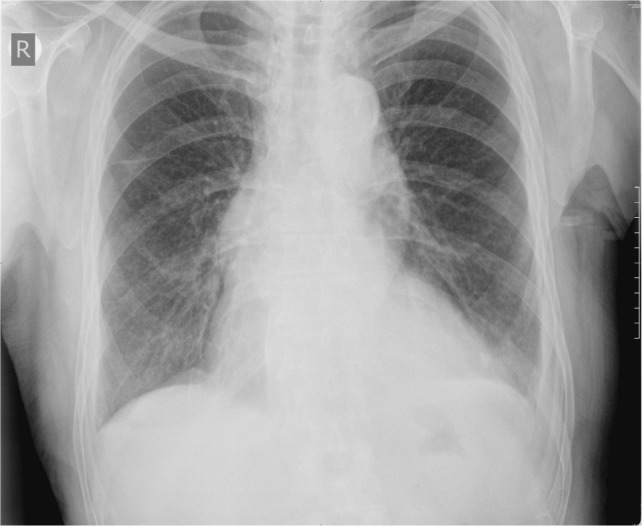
Chest radiography after 3 months of treatment.
